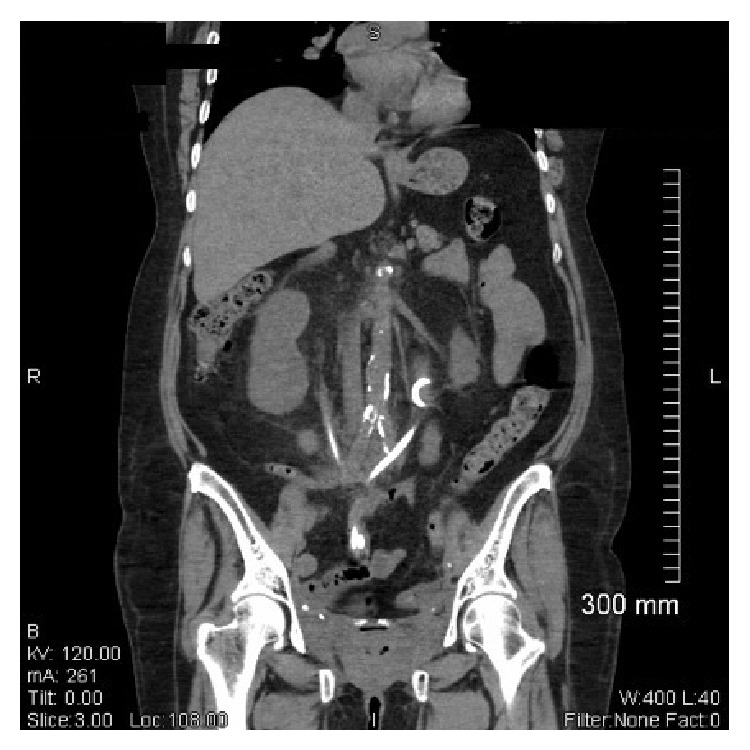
Sagittal view of patient's CT scan, without contrast, showing mild bilateral hydronephrosis and proper stent placement through the conduit with no evidence of pseudoaneurysm of the distal aorta or iliac vessels.
